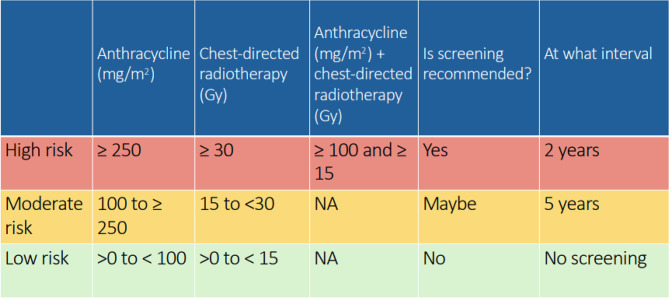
Recommended frequency of echocardiogram
